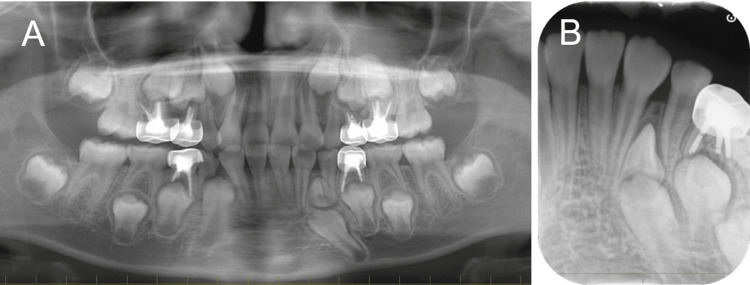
Re-evaluation at the age of eight years and three months (A) Panoramic radiograph; (B) Periapical photograph
